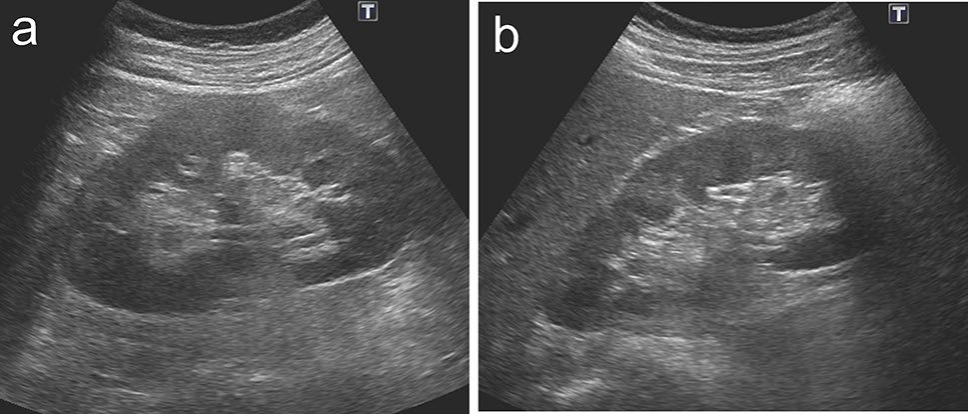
Abdominal ultrasound findings at the time of the initial renal biopsy. a, b No mass lesions were observed in the left kidney (a) and the right kidney (b)
